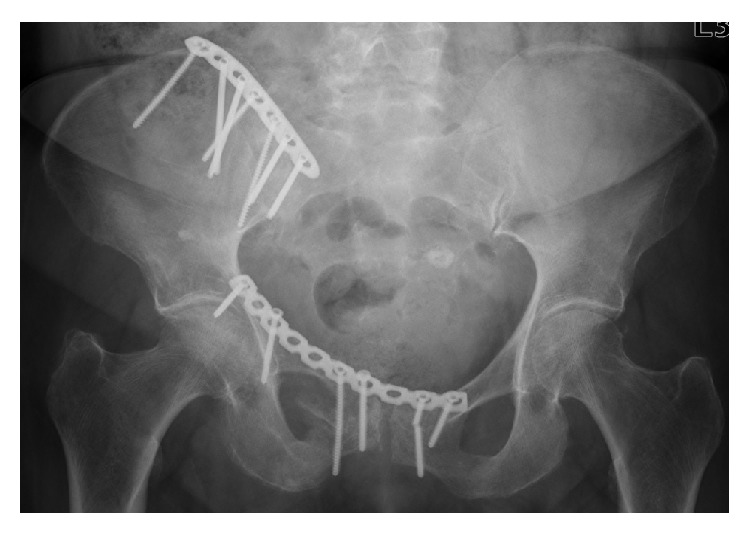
Postoperative X-ray 3 months after surgery.
